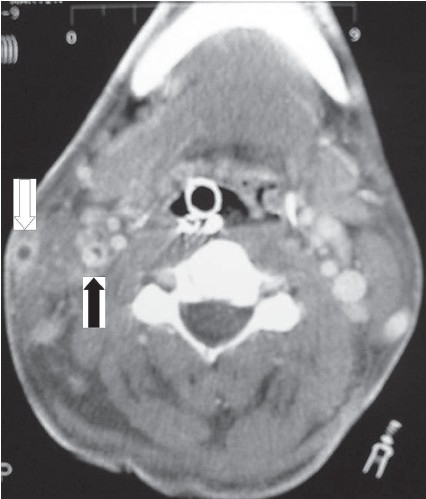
CT of the neck showing partial thrombosis of the right internal jugular vein (black arrow) and complete thrombosis of the right external jugular vein (white arrow).
